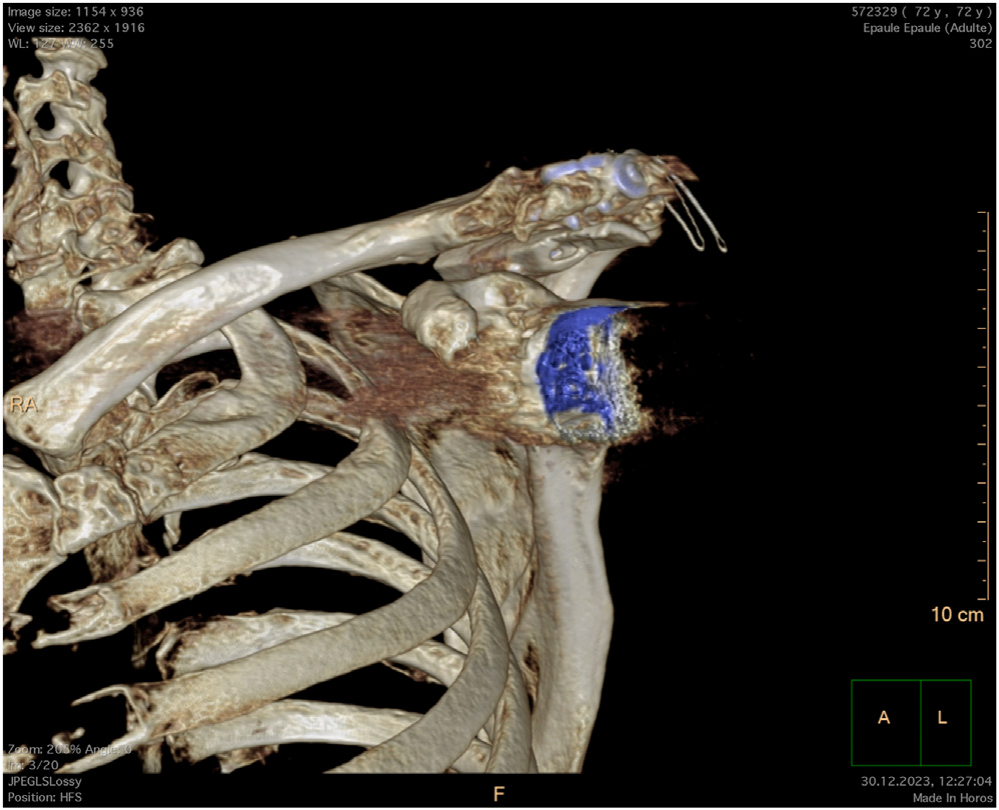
Postoperative 3-dimensional computed tomography reconstruction of a left shoulder with glenosphere and humerus subtraction. Note the fibular allograft reinforcement anchored in the supraspinous fossa to support the undersurface of the acromion.
